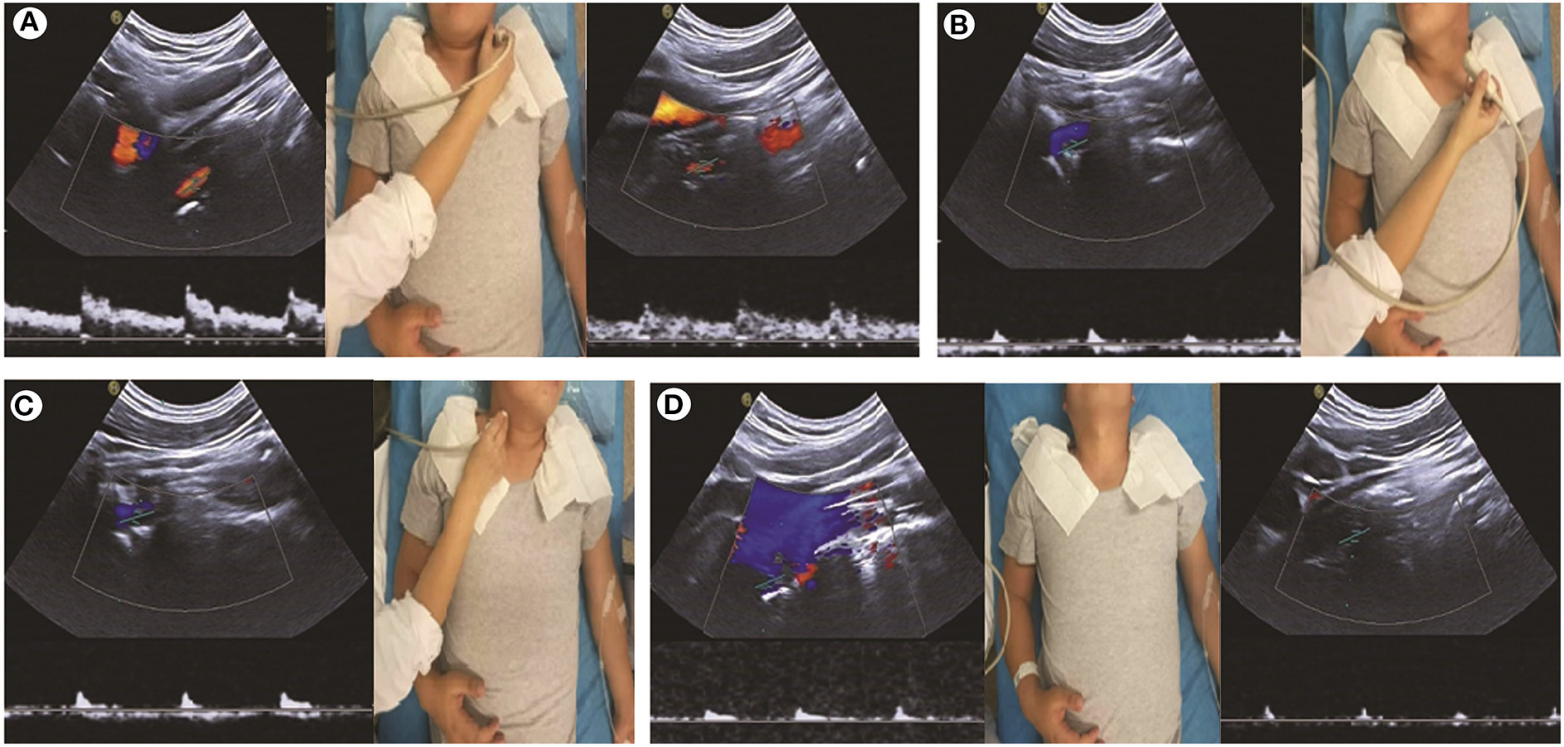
Carotid artery ultrasound shows abnormalities during neck rotation. (A) The velocity of blood flow and spectrum of the bilateral vertebral artery (VA) is normal with the patient's neck in the neutral position. (B, C) Low bloodstream and high resistance during the head rotation to the contralateral side, indicating that the distal part of the VA is occluded. (D) Bilateral VA is occluded when the head is tilted backward.
